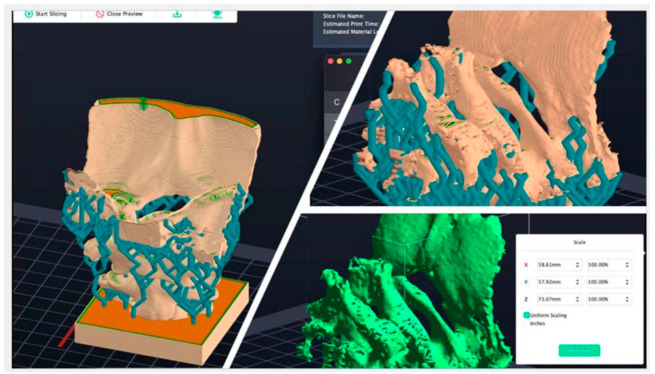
Three-dimensional printing reconstruction model of the cervical spine derived from CT imaging data.
